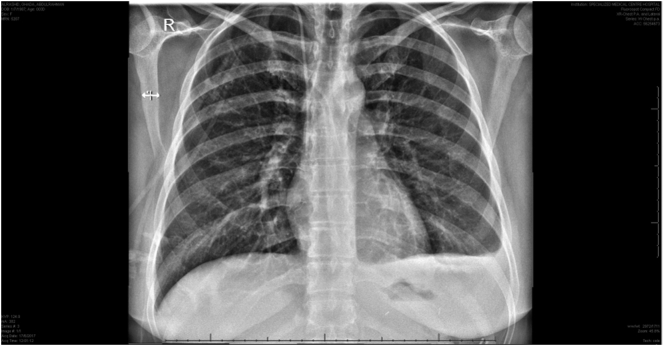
Follow up chest X-ray performed two days after POSE reversal, revealing absence of pneumoperitoneum, decreased left-sided pleural effusion (though still present), and clear lungs.
